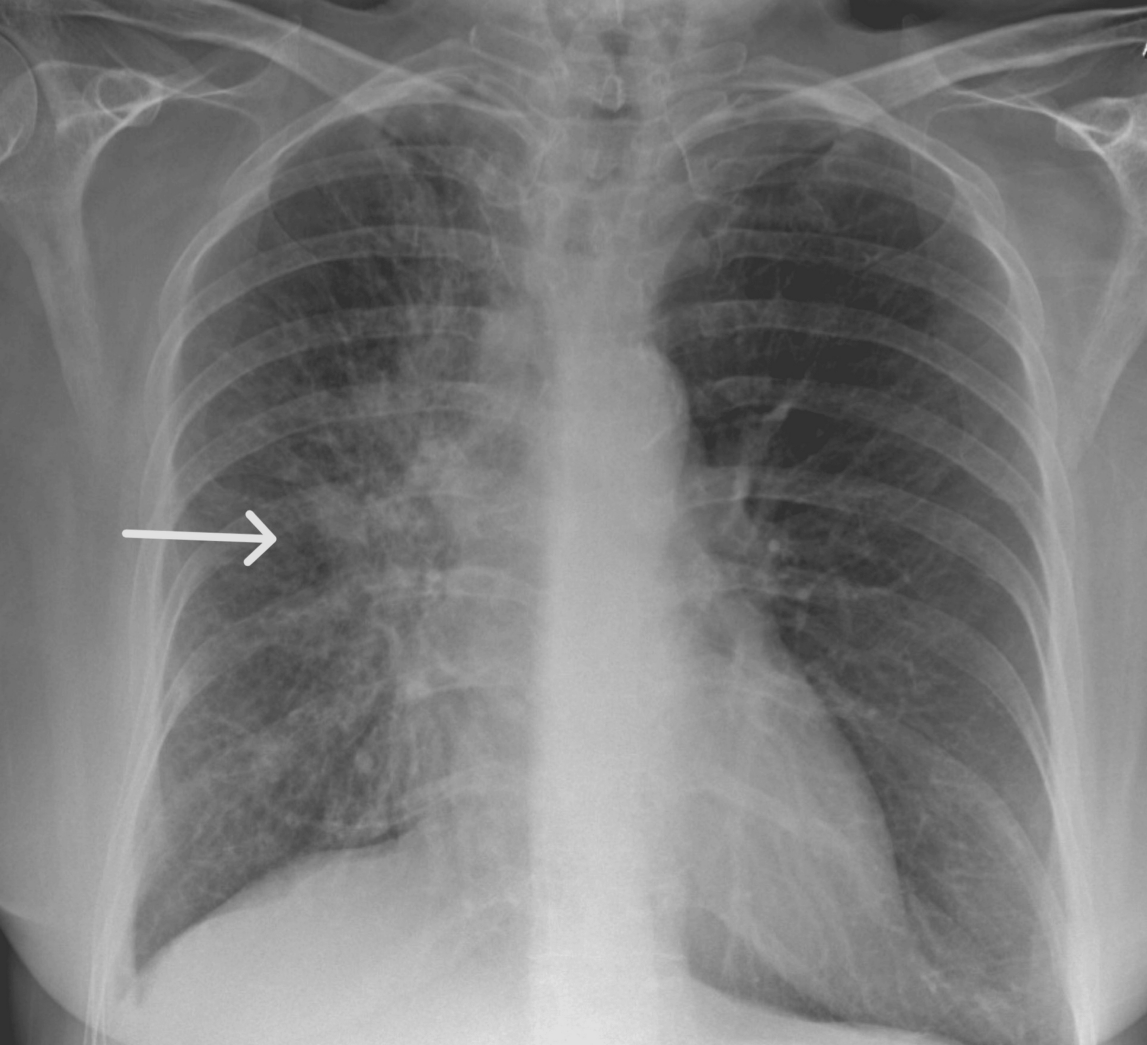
Initial chest X-ray showing a bulky right hilum
